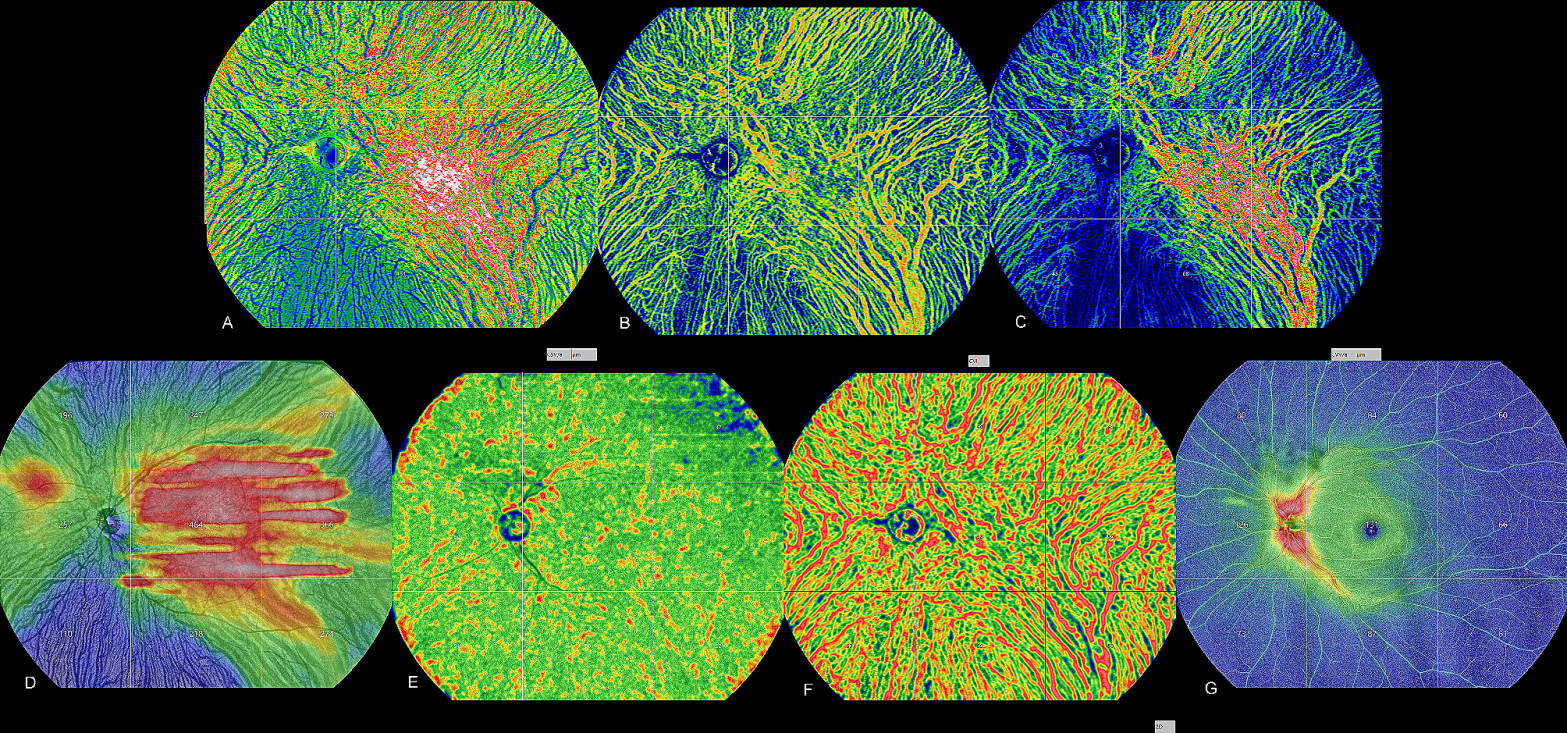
Ultra-widefield swept-source optical coherence tomography (UWF SS-OCTA) images that are representative (A) Choroid vessel volume per unit area (mCVV/a) across nine subfields (B) Three-dimensional choroidal vessel volume index (3D-CVI) across nine subfields (c) Choroid stroma volume per unit area (mCSV/a) across nine subfields (D) Choroidal thickness (CT) across nine subfields (E) Vascular density of the choriocapillaris layer across nine subfields (F) Vascular density of the large choroidal vesasel layer across nine subfields (G) Retinal thickness (RT)
